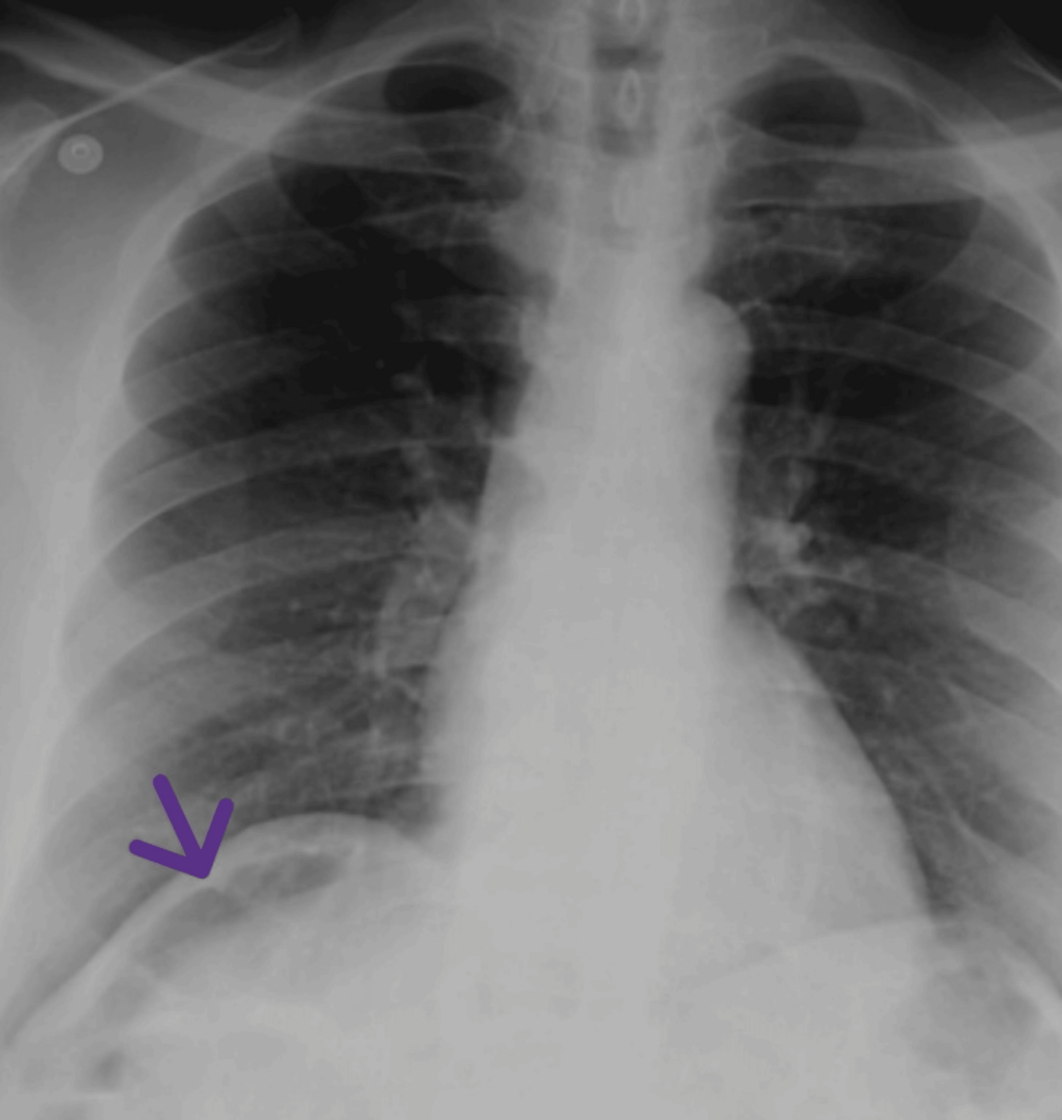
Erect X-ray denoting Chilaiditi sign (pseudopneumoperitoneum) mimicking apparent free intraperitoneal air under the right hemidiaphragm (purple arrow)
